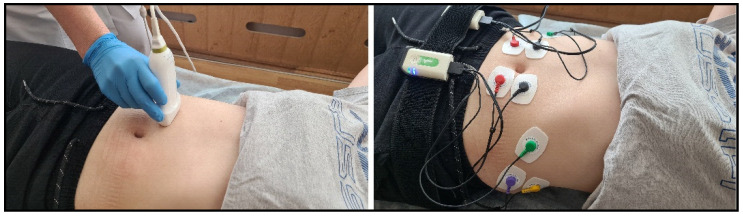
Ultrasound examination of muscles (left) and EMG examination (right).
